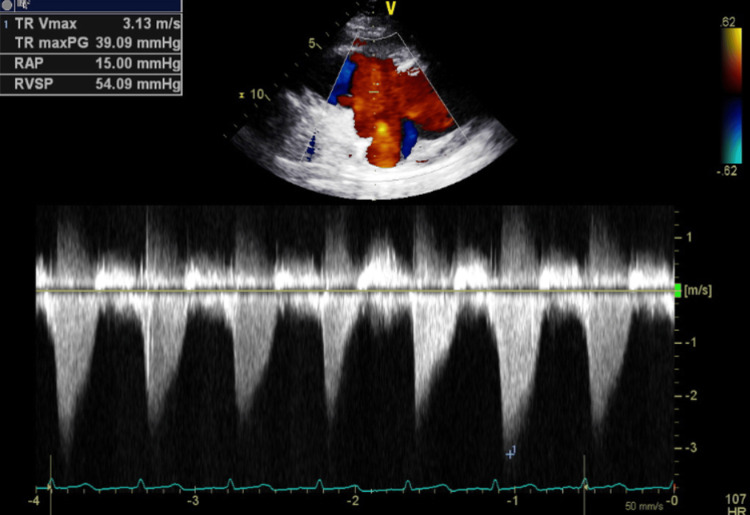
Transthoracic echocardiography post-pericardiocentesis - image 5 Transthoracic echocardiography post-pericardiocentesis; apical 4-chamber view measuring TR Vmax at 3.13 m/s, RAP at 15 mmHg, and RVSP at 54.09 mmHg, indicative of elevated right side pressure
